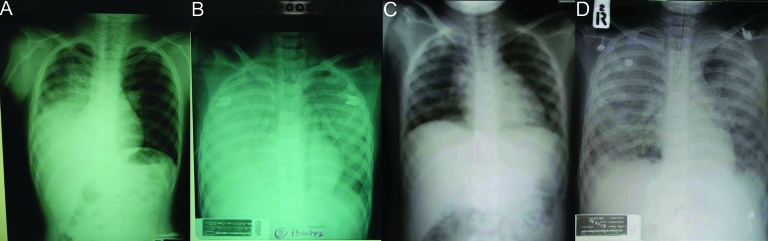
Chest radiographs from patients 8 and 9. Panel A demonstrates patchy alveolar infiltration of the right lower lung on day 5 of illness for patient 9; panel B demonstrates the progression to acute respiratory disease syndrome (ARDS) on day 8. Panel C shows interstitial infiltration of both lungs of patient 8 on day 4 of illness; panel D shows the rapid progression to ARDS by day 6.
